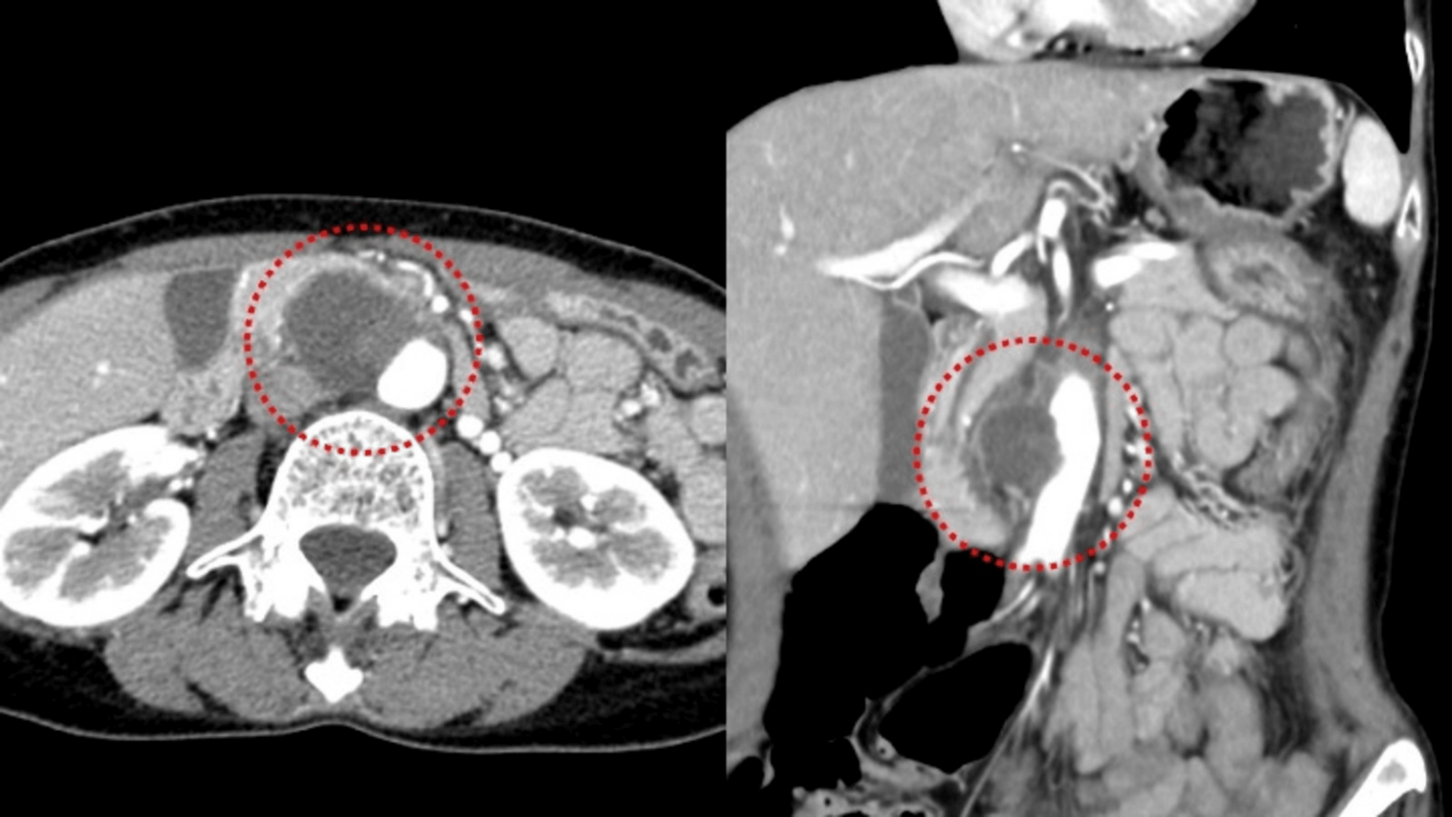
Preoperative contrast-enhanced CT The red dashed line encloses the abdominal aortic aneurysm, which was initially diagnosed as an impending rupture based on clinical presentation and imaging findings.
